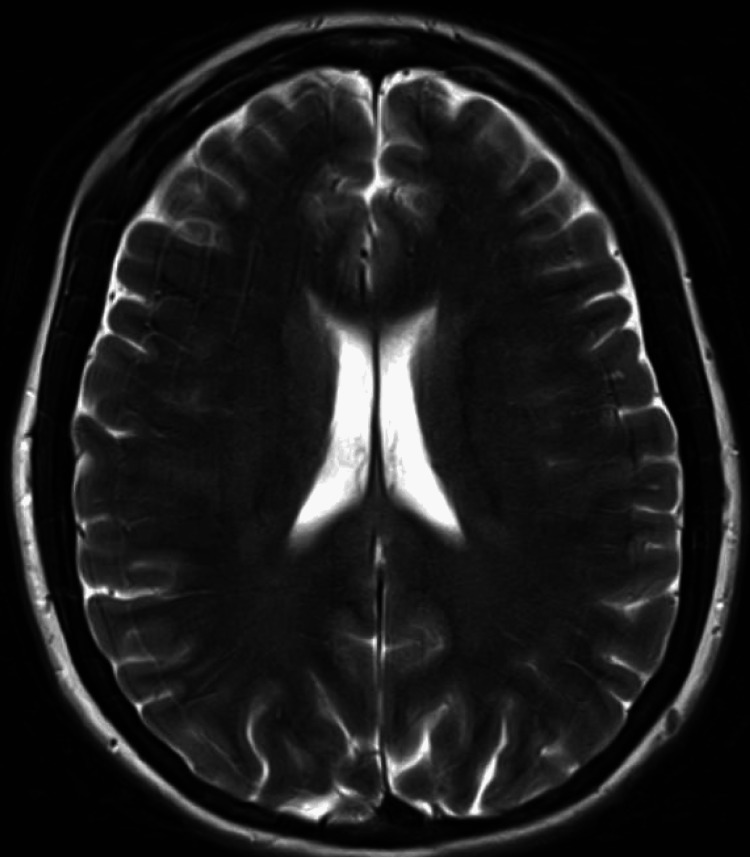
Axial T2-Weighted Sequence MRI of the Brain Axial T2-weighted MRI of the brain showing no acute pathology, with no significant changes compared to prior imaging.
